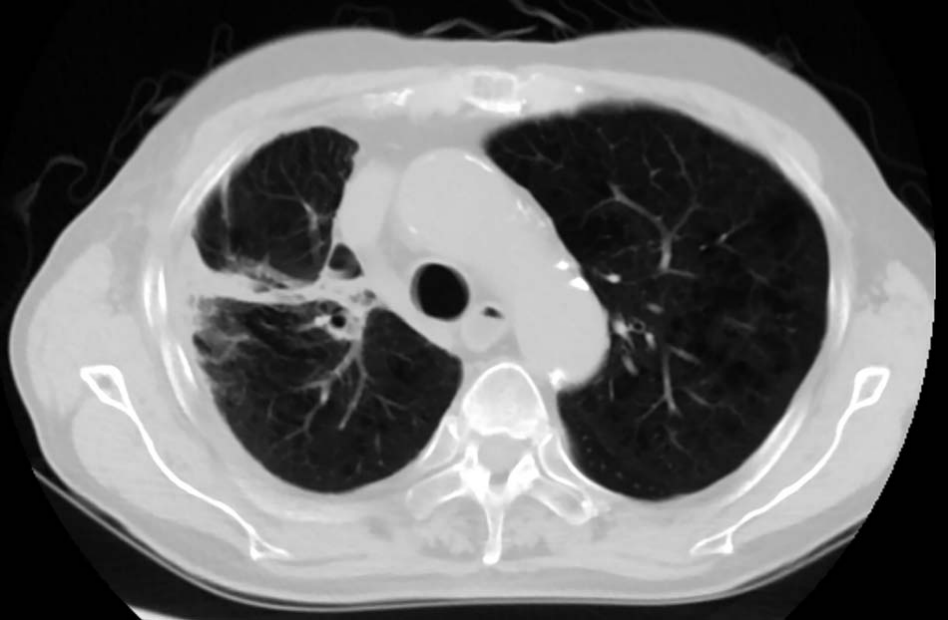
Follow-up computed tomography image of the same patient shown in Figure 1. Imaging was acquired 6 months from treatment. There was no evidence of recurrence and out-of-irradiated-field pneumonitis.
